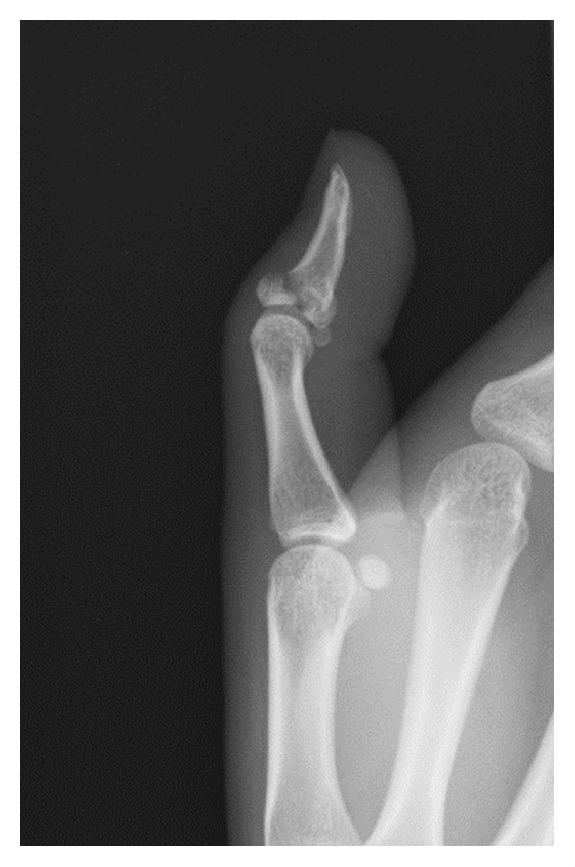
The plain radiograph shows a bony mallet thumb.
